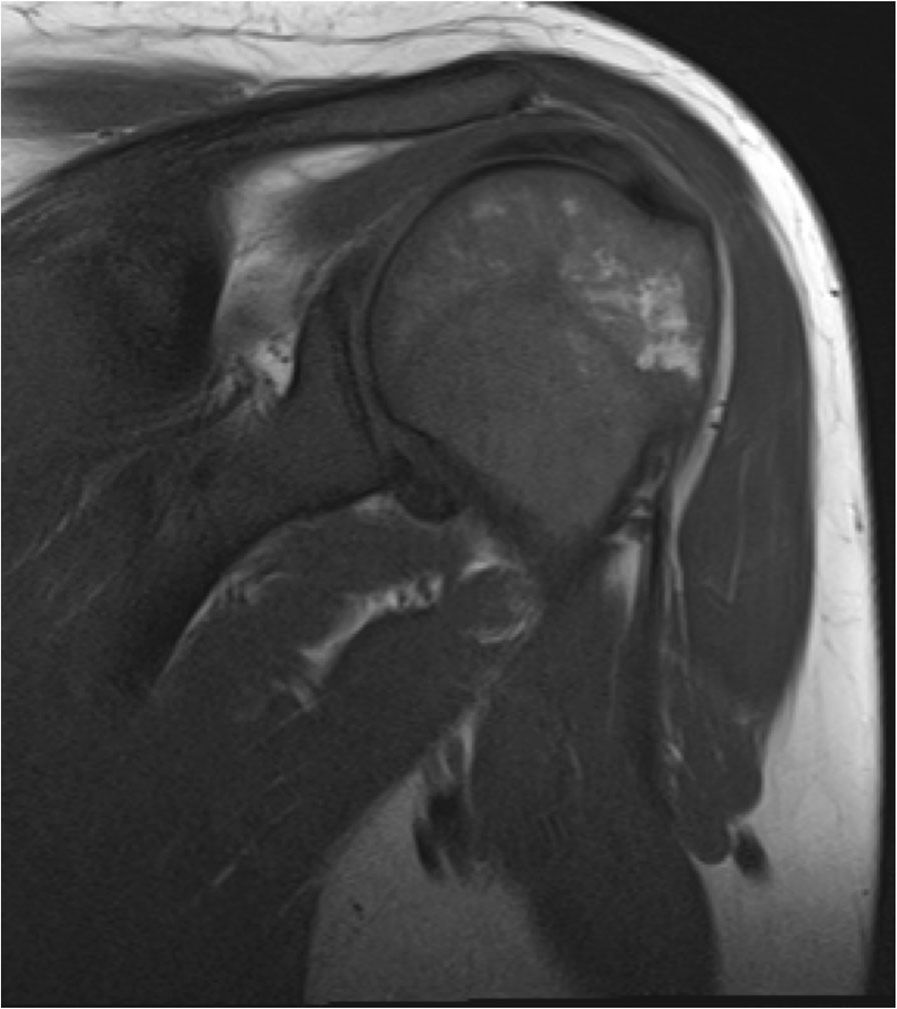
Marrow infiltration in Gaucher disease. A 30-year-old female with type 1 Gaucher disease with shoulder pain. Coronal T1-weighted imaging demonstrates diffuse hypointense infiltration of the humerus including the proximal epiphysis compared to subcutaneous fat
